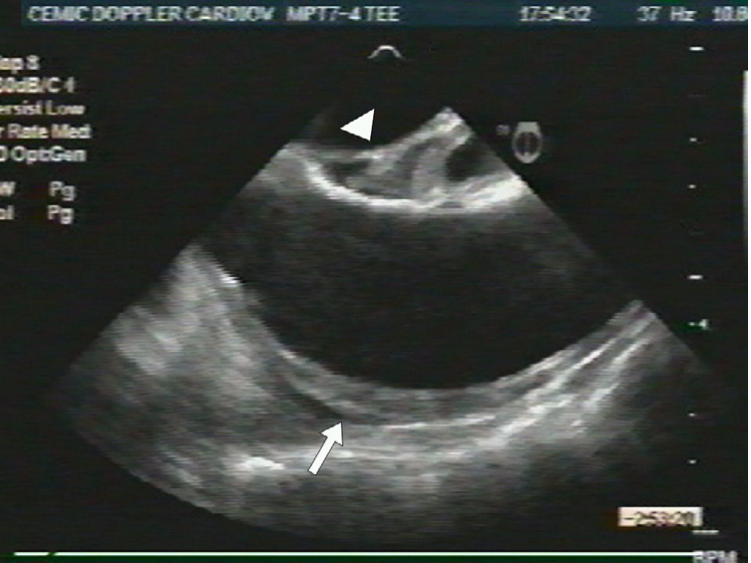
Transesophageal echocardiogram in the longitudinal plane showing aortic intramural hematoma compromising its anterior aspect (arrow). Notice the partially organized extravasated blood occupying the Theile sinus (arrowhead).
